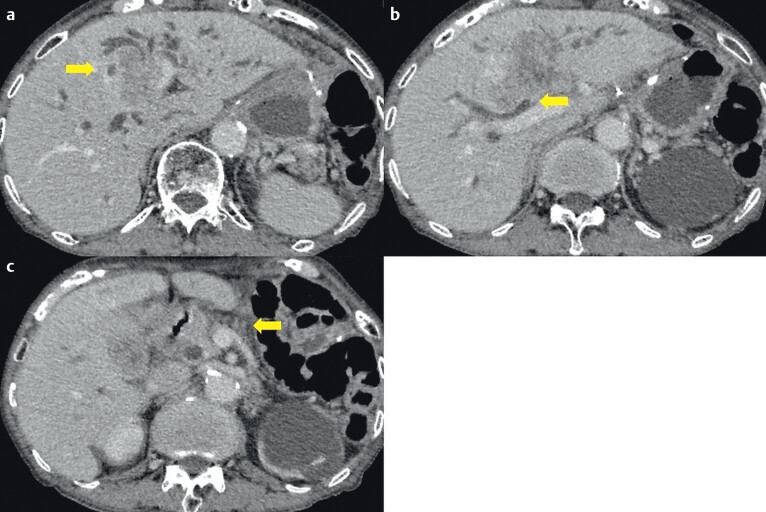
Preprocedural contrast-enhanced computed tomography. (
